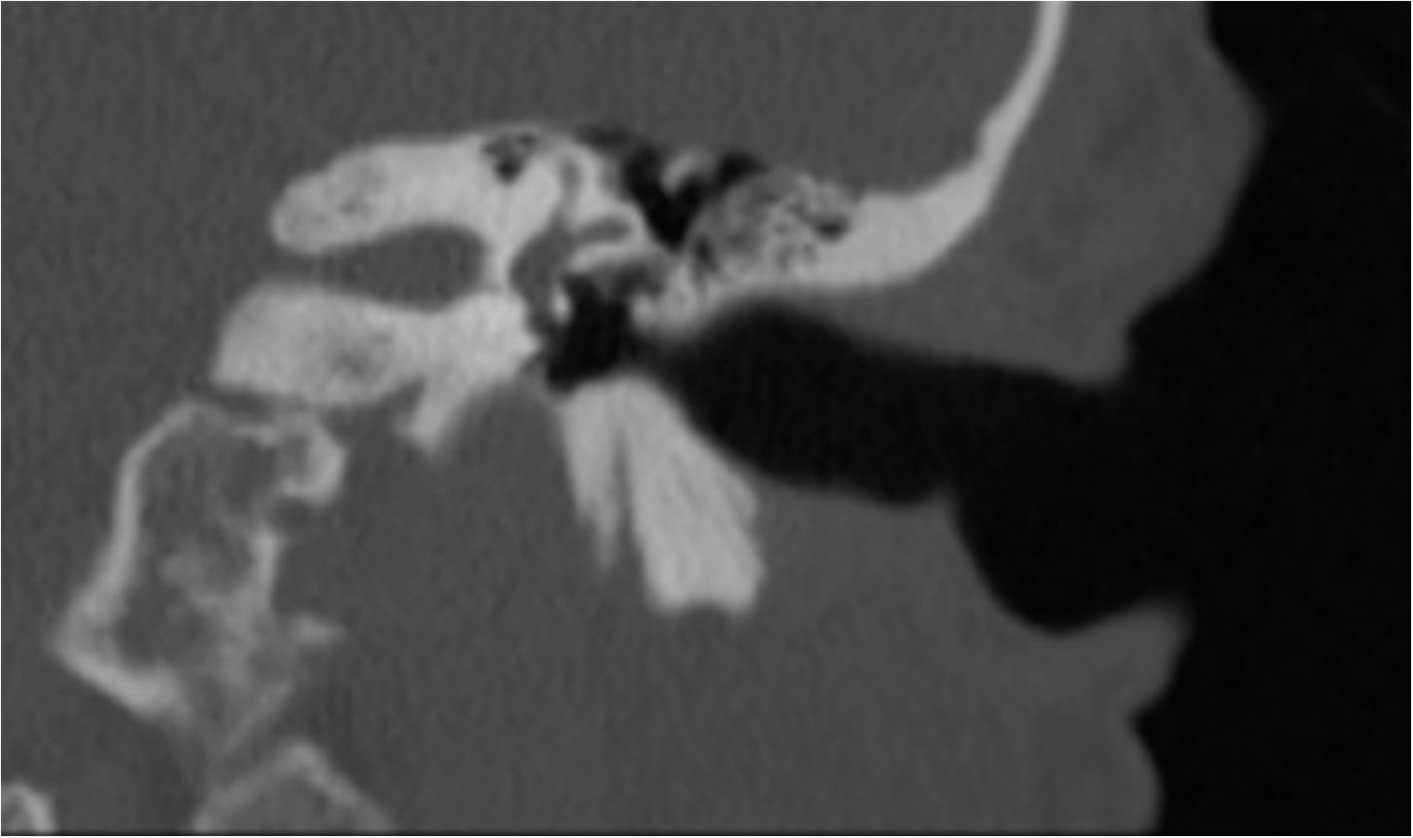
Coronal CT of temporal bone of the same patient in Figure 3 after perilymph fistula repair procedure. No air is seen in the inner ear.
